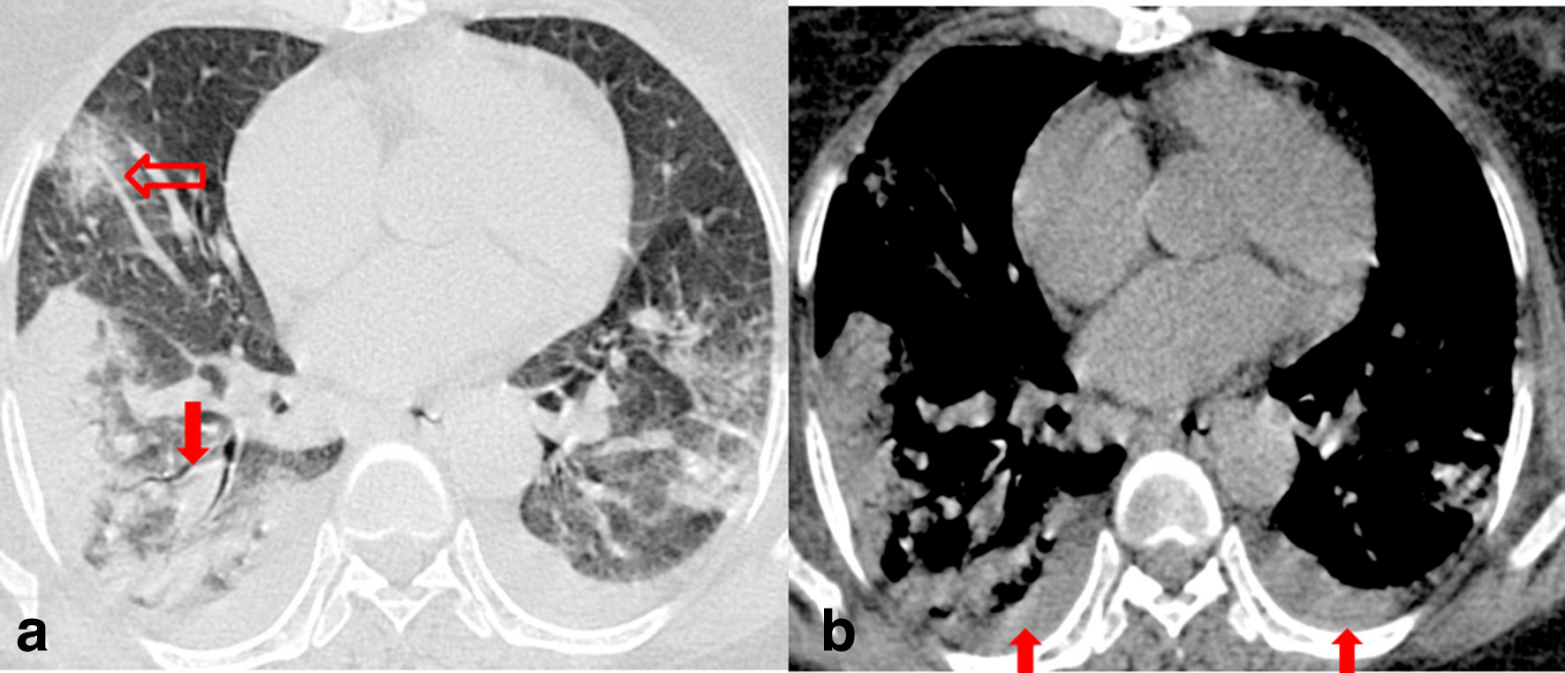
Non-contrast axial chest CT image (a) in lung window settings of a 60-year-old COVID-19 positive female patient, admitted in the ICU (clinically unstable), showing bilateral GGOs with consolidation on the right side. Air bronchogram sign is denoted by the solid arrow and vessel dilatation by a hollow arrow in (a). Axial CT image in the mediastinal window (b) shows bilateral mild pleural effusion denoted by arrows in (b). COVID-19, Corona virus disease 2019; GGO, ground glass opacity; ICU, intensive care unit.
